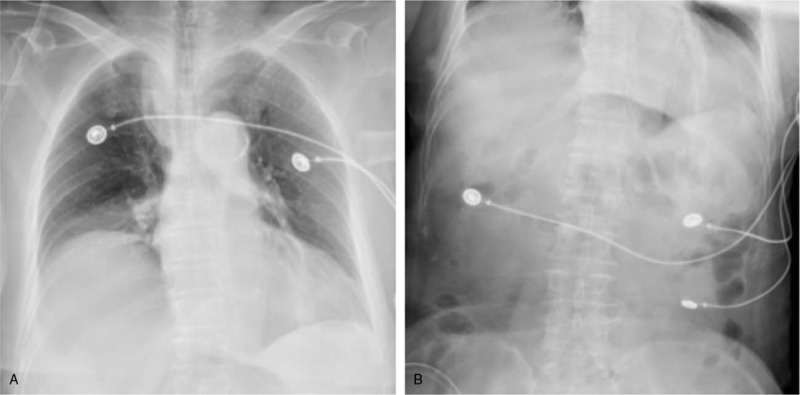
Chest (A) and semi-supine abdominal plain (B) radiographs showing enhanced lung markings, shadows in the left lower lung lobes, elevation of the right diaphragm, and mild pneumoperitoneum.
